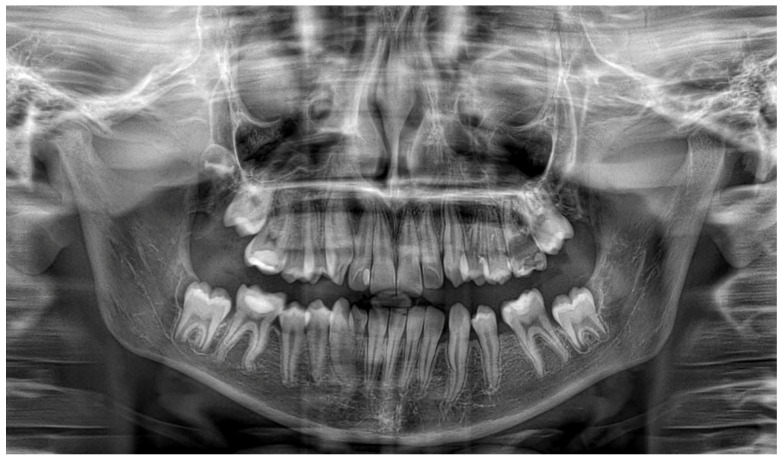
A three-year follow-up panoramic radiograph showing full resolution of the pathologies.
